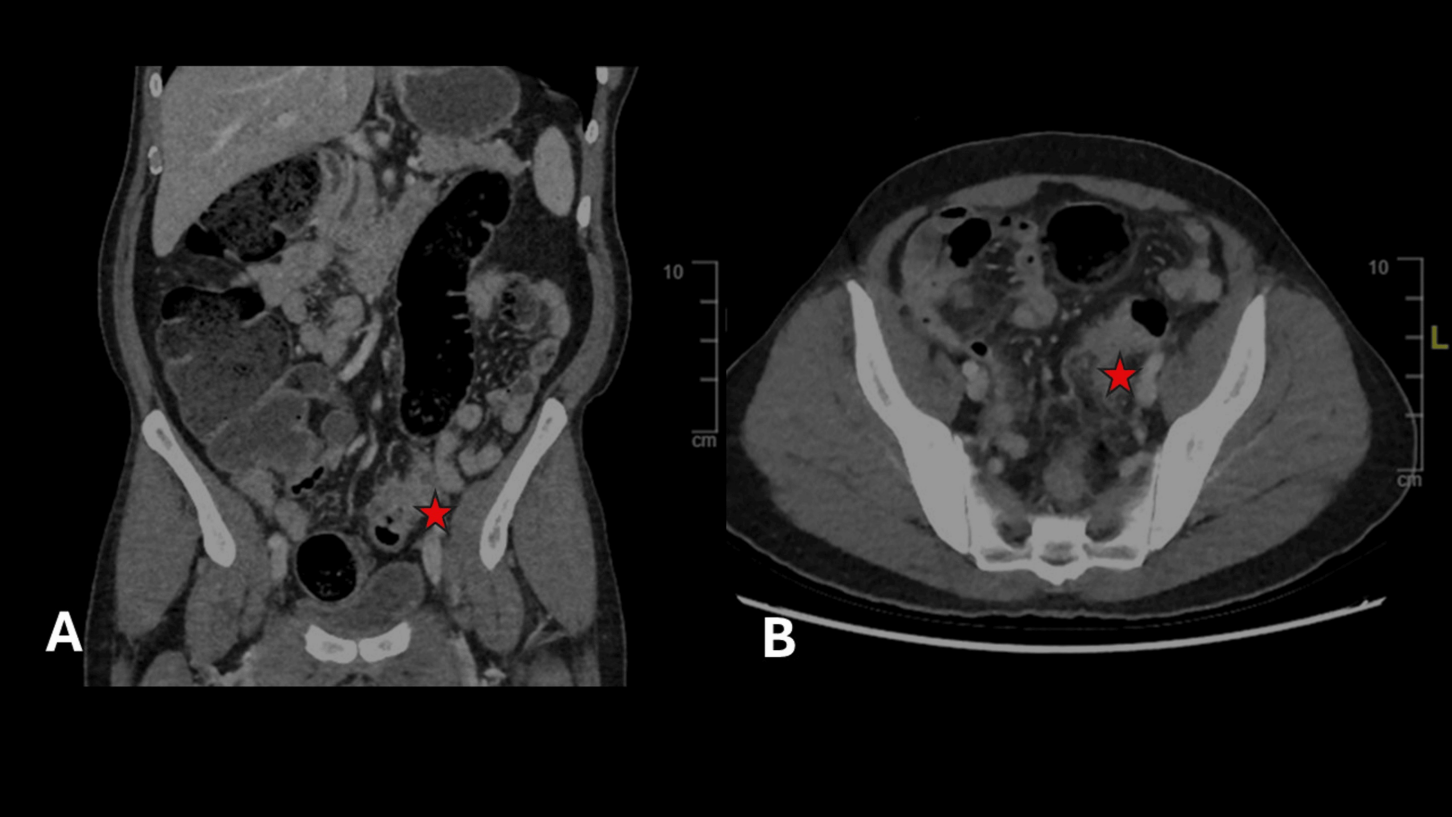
Abdominal CT showing sigmoid colon mass. (A) Coronal section. (B) Axial section
